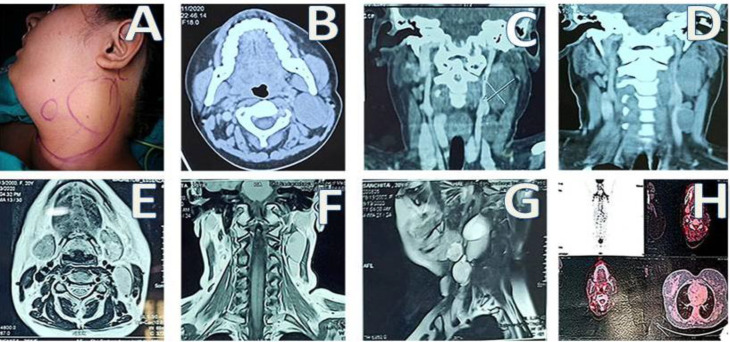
A: Two discrete swellings in the neck; B, C, D: CECT neck showed iso to hypodense lesions measuring 3.3x2.7x5.9cm at level II and III on the left side, E, F, G: MRI neck, H: 18F-FDG whole-body PET CT scan
